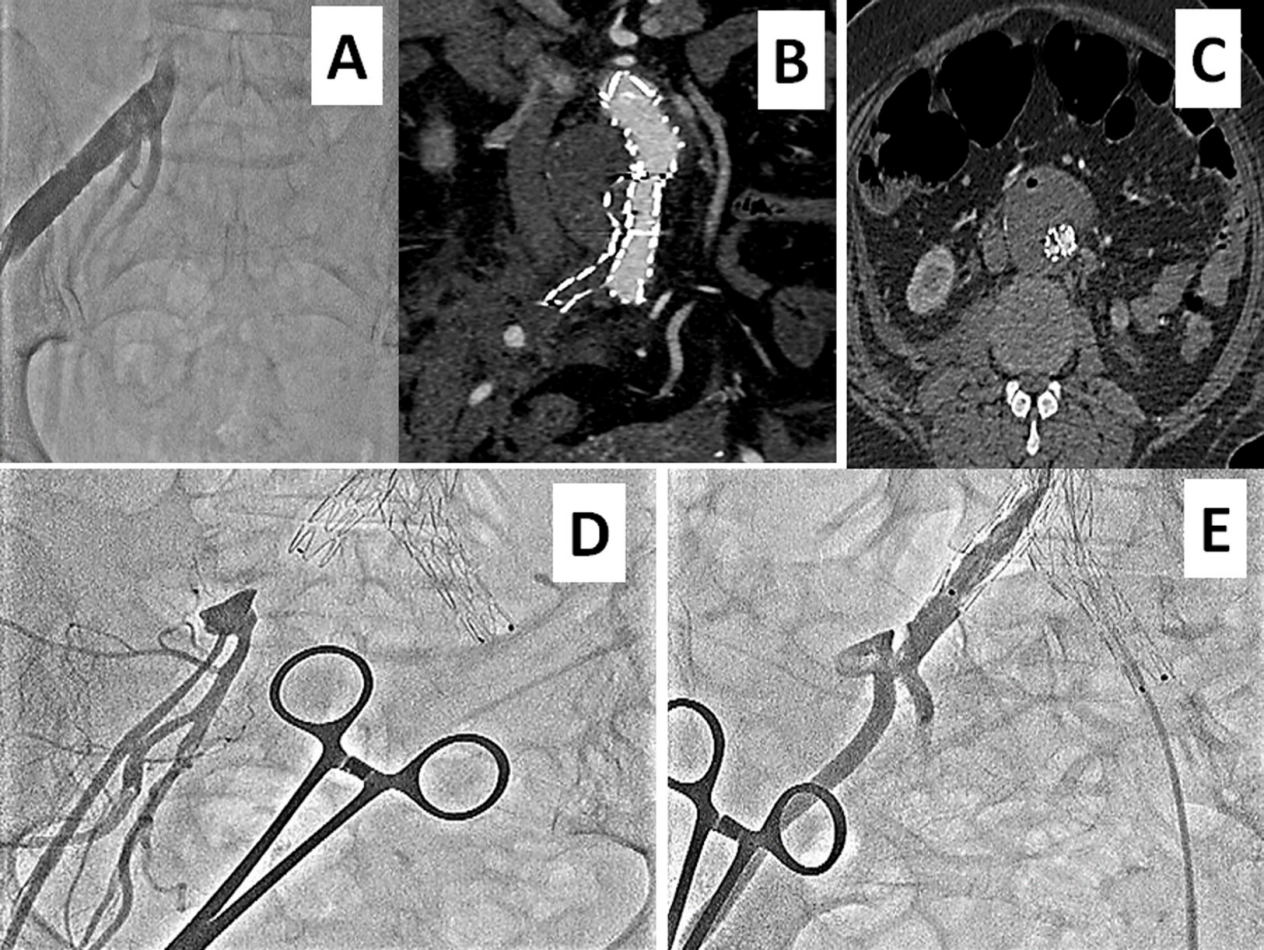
A PTFE conduit was anastomosed to the right CIA (A) in abdominal aortic aneurysm (AAA) case 4. Post-intervention, day-3 CT reconstructed coronal and axial images showed thrombotic occlusion of the right limb of the aortic stent graft (B & C). Following balloon angioplasty and thrombus suction (D), good flow was achieved across the occluded right limb (E).
